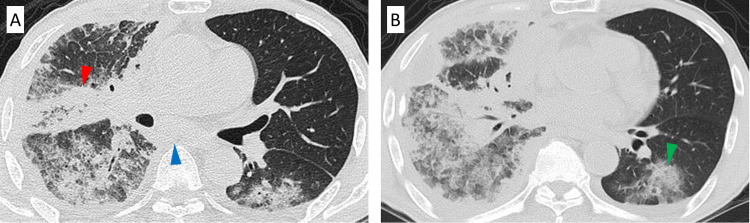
Chest computed tomography on day 17 after initiation of treatment with pembrolizumab Chest computed tomography on day 17 after treatment initiation with pembrolizumab showed that the tumor size had increased (red arrow and blue arrow) (A), the pleural effusion had worsened, and new ground-glass shadows appeared in the left lung (green arrow) (B).
